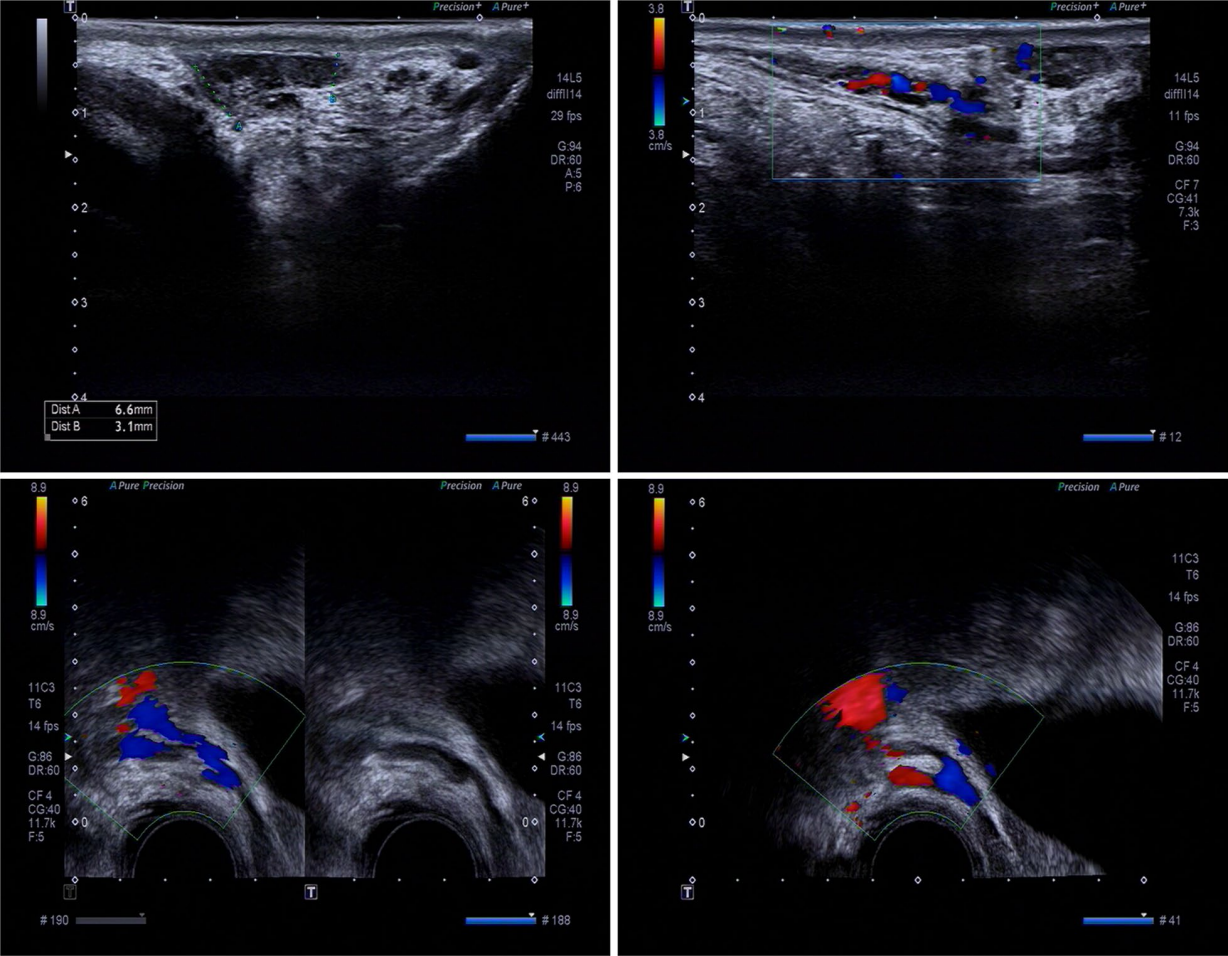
Scrotal and transrectal ultrasonography revealed the absence of the tail of epididymide, vas deferens and seminal vesicle on the right side, and dysplastic seminal vesicle on the left side
